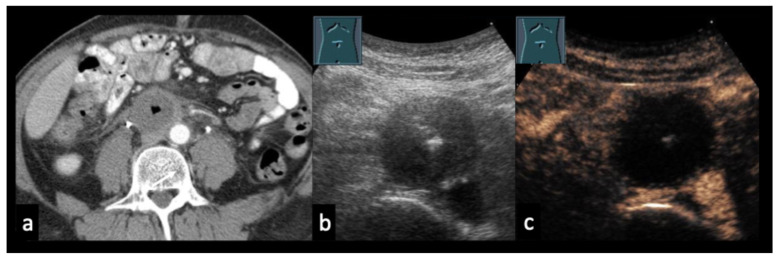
(a,b) 48-year-old patient with testicular carcinoma and paraaortic lymph node metastases after four cycles of chemotherapy. CT ((a), arrow) and B-mode ultrasound (b) demonstrate a hypoechoic residual lymph node; β-HCG remained mildly elevated at 16 U/L. (c): CEUS at 39 s shows absence of enhancement, indicating predominantly necrotic tissue. A residual tumor resection was planned; however, a paraaortic local recurrence developed prior to the scheduled surgery.
